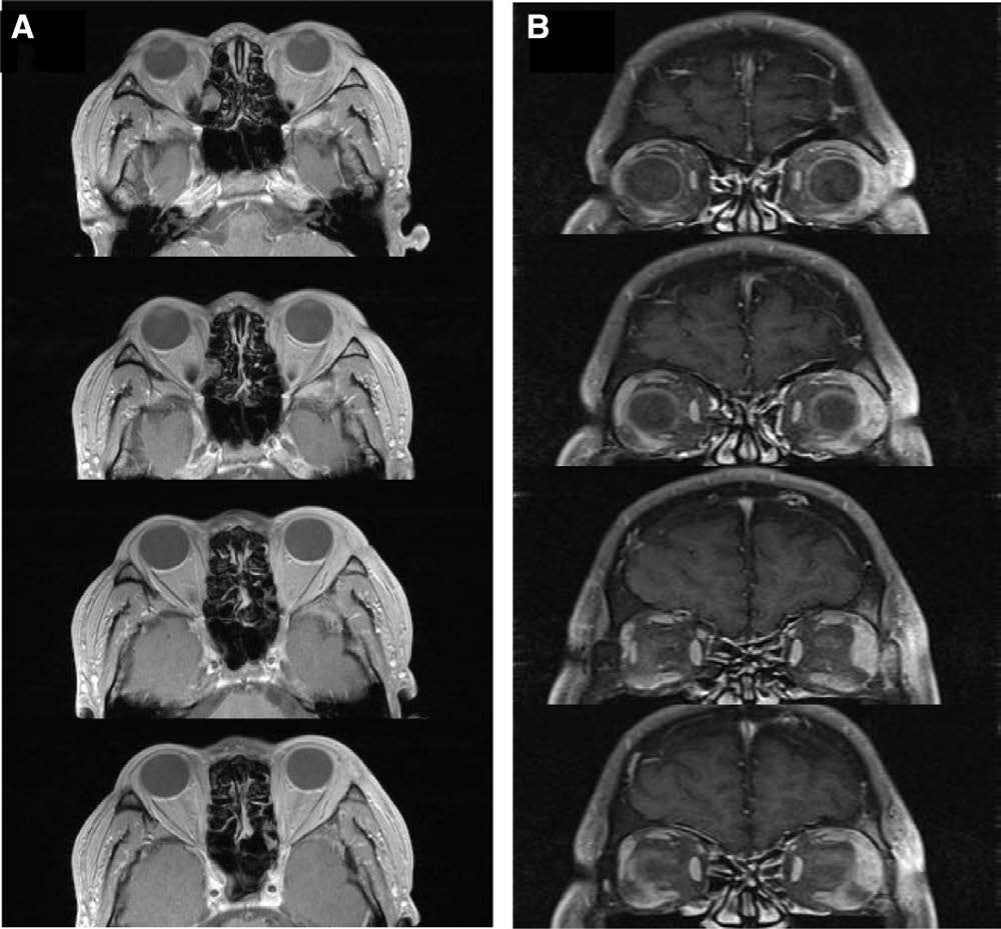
Axial (A) and coronal (B) gadobutrol-enhanced T1-weighted magnetic resonance image showing a left orbital mass in the area of the lacrimal gland (bony wall of orbit was infiltrated, the periosteum was intact).
